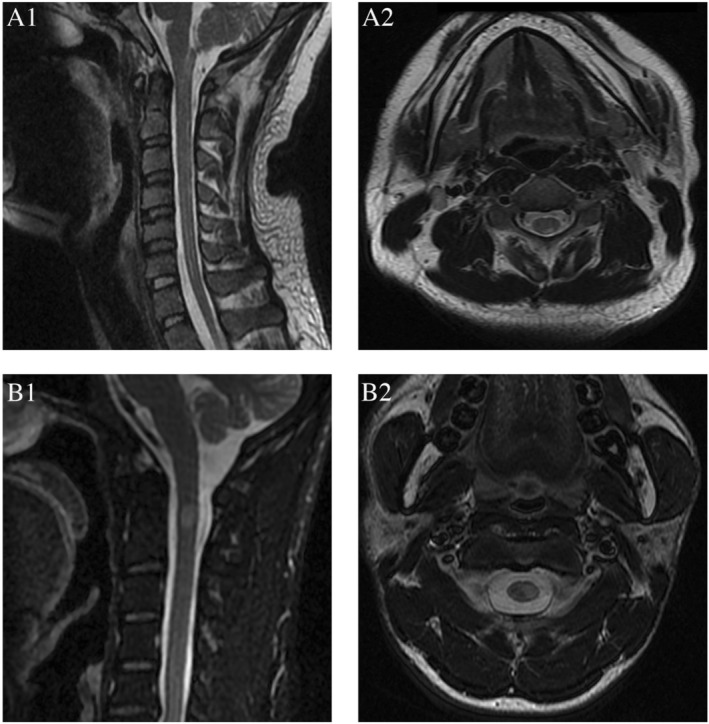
The eccentric signs of SCI on axial spinal MRI. The eccentric signs of SCI of a SCI patient on the sagittal (A1) and the axial (A2) spinal MRI are compared with an ITM patient (B1–B2). ITM, idiopathic transverse myelitis; MRI, magnetic resonance imaging; SCI, spinal cord infarction.
